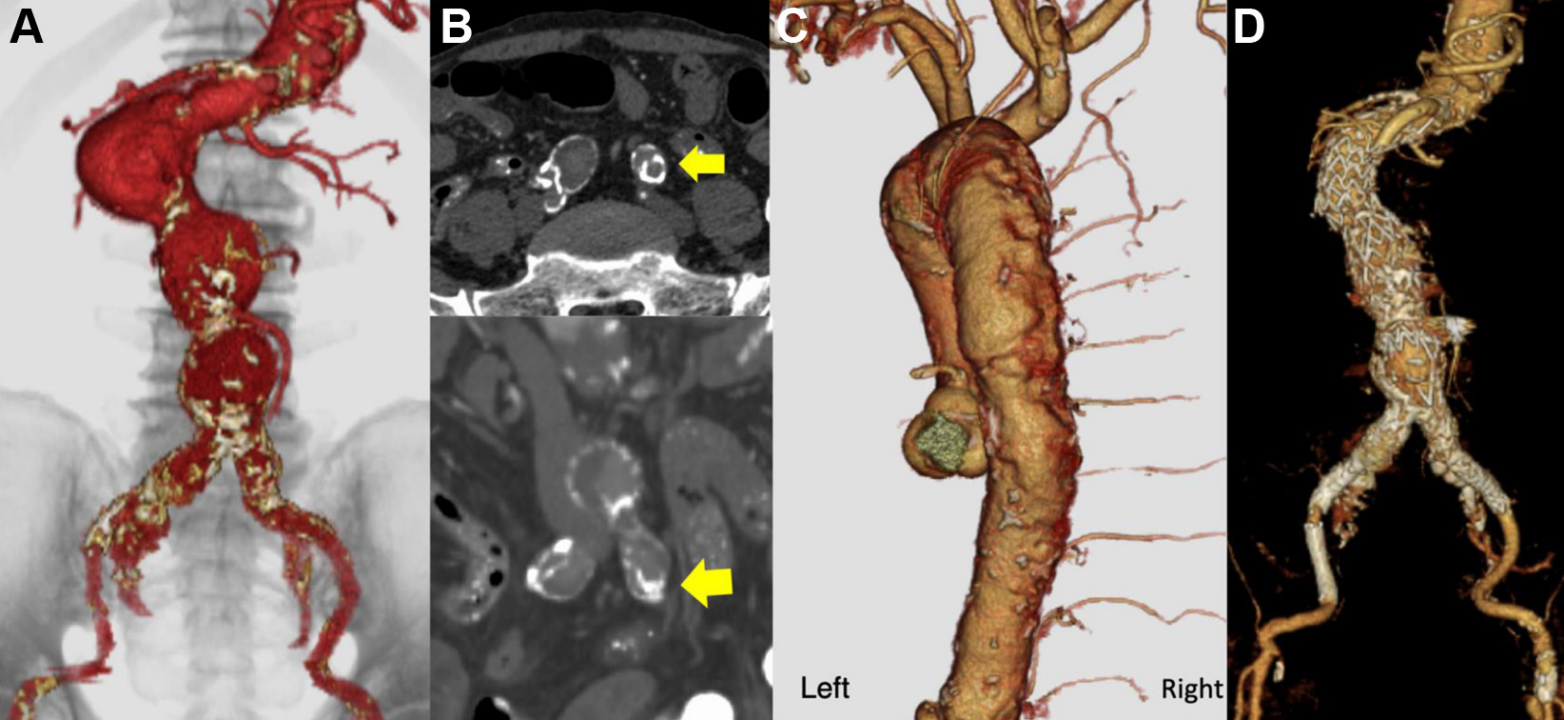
Preoperative and postoperative enhanced computed tomography (CT) findings. (A) Abdominal aortic aneurysm (AAA) with three humps. (B) Axial (upper) and coronal (lower) CT scan showed the left common iliac artery (CIA) (yellow arrows) with heavily calcification and partial dissection. (C) Multiple occlusions of the left intercostal arteries between Th4 and Th12 owing to previous aortic dissection. (D) Blood flow in the lower extremities after endovascular AAA repair was confirmed.
